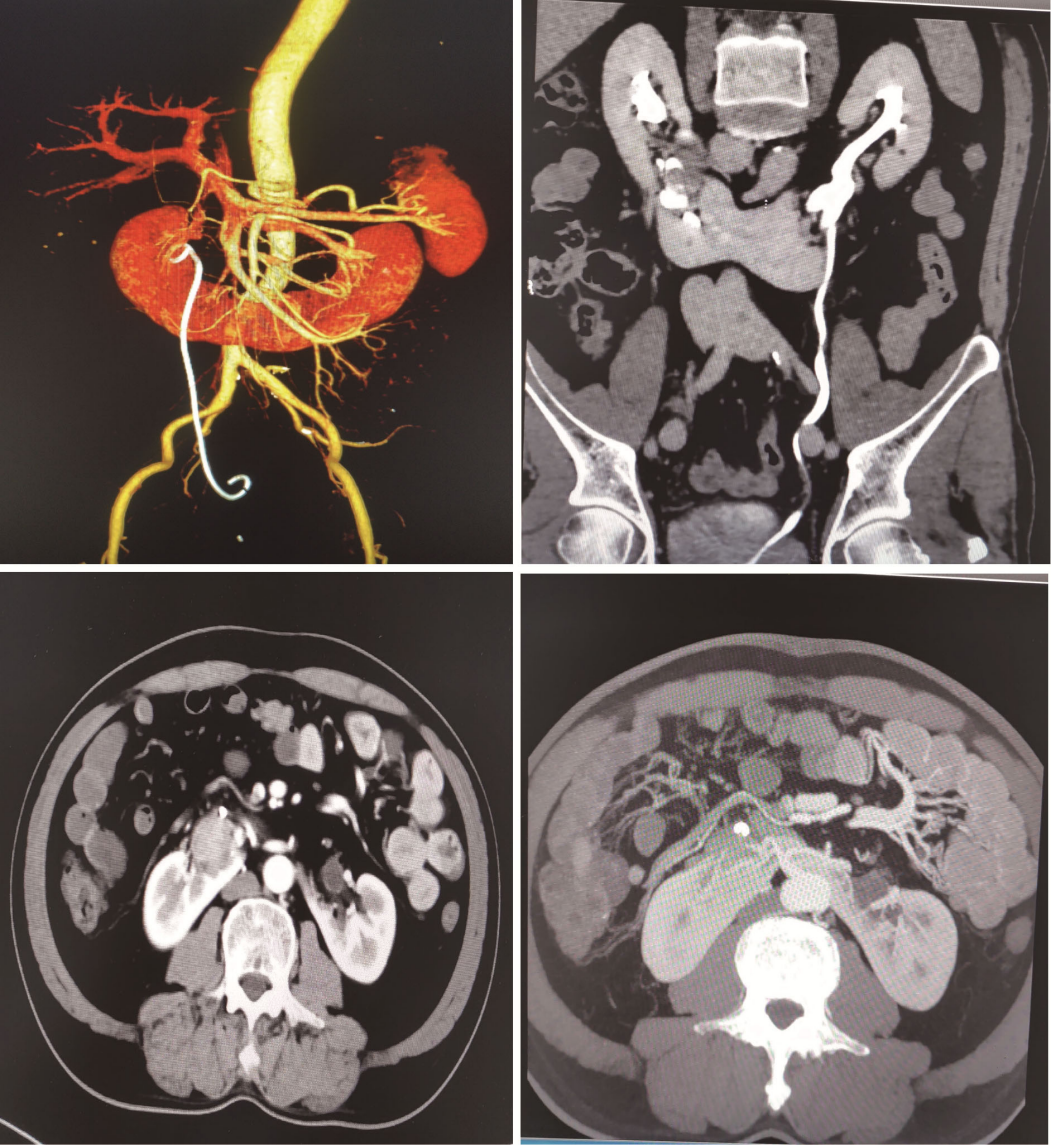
CTU and CTA show well-defined right renal pelvis mass complicated with horseshoe kidney and hydronephrosis.
